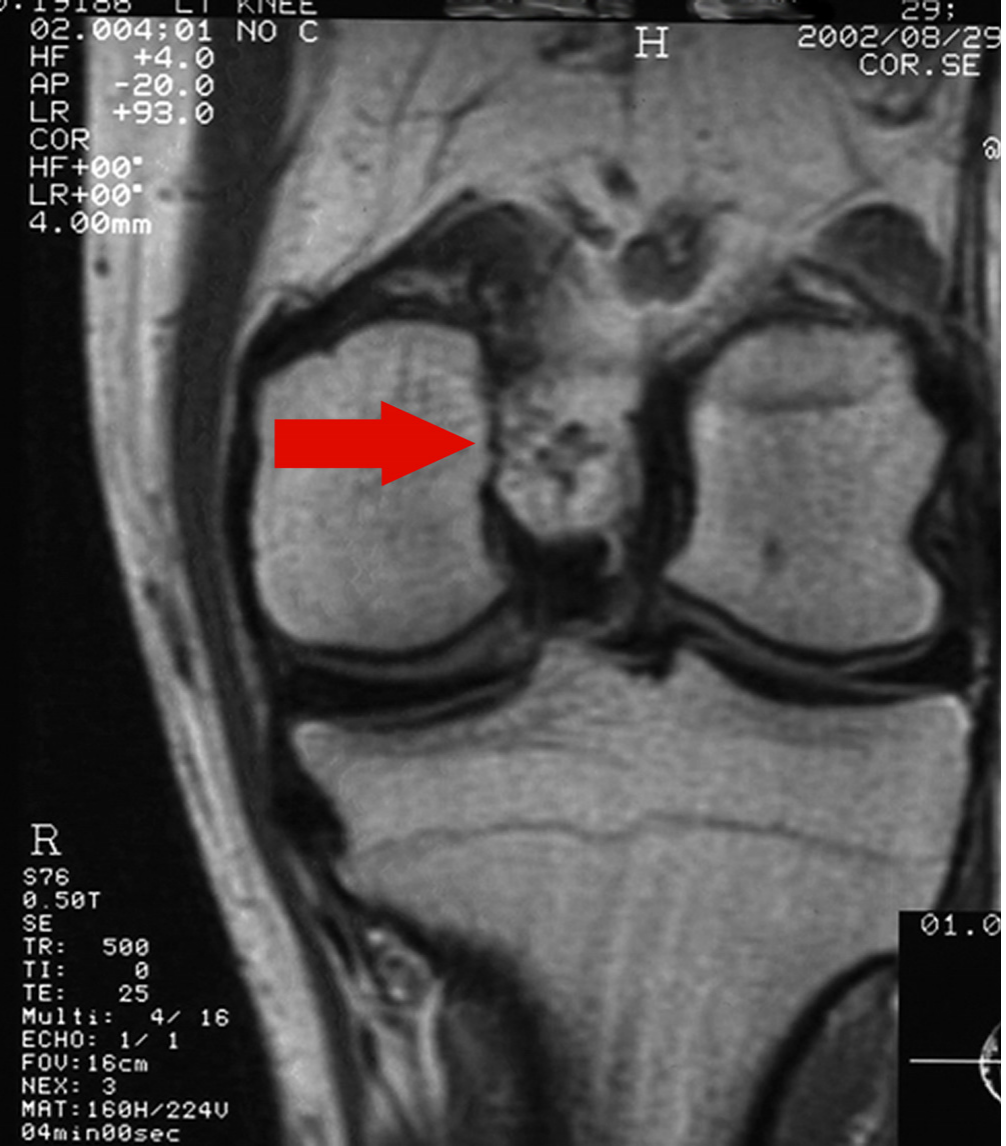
Magnetic resonance imaging showing a haemangioma located in the femoral inter-condylar notch.
